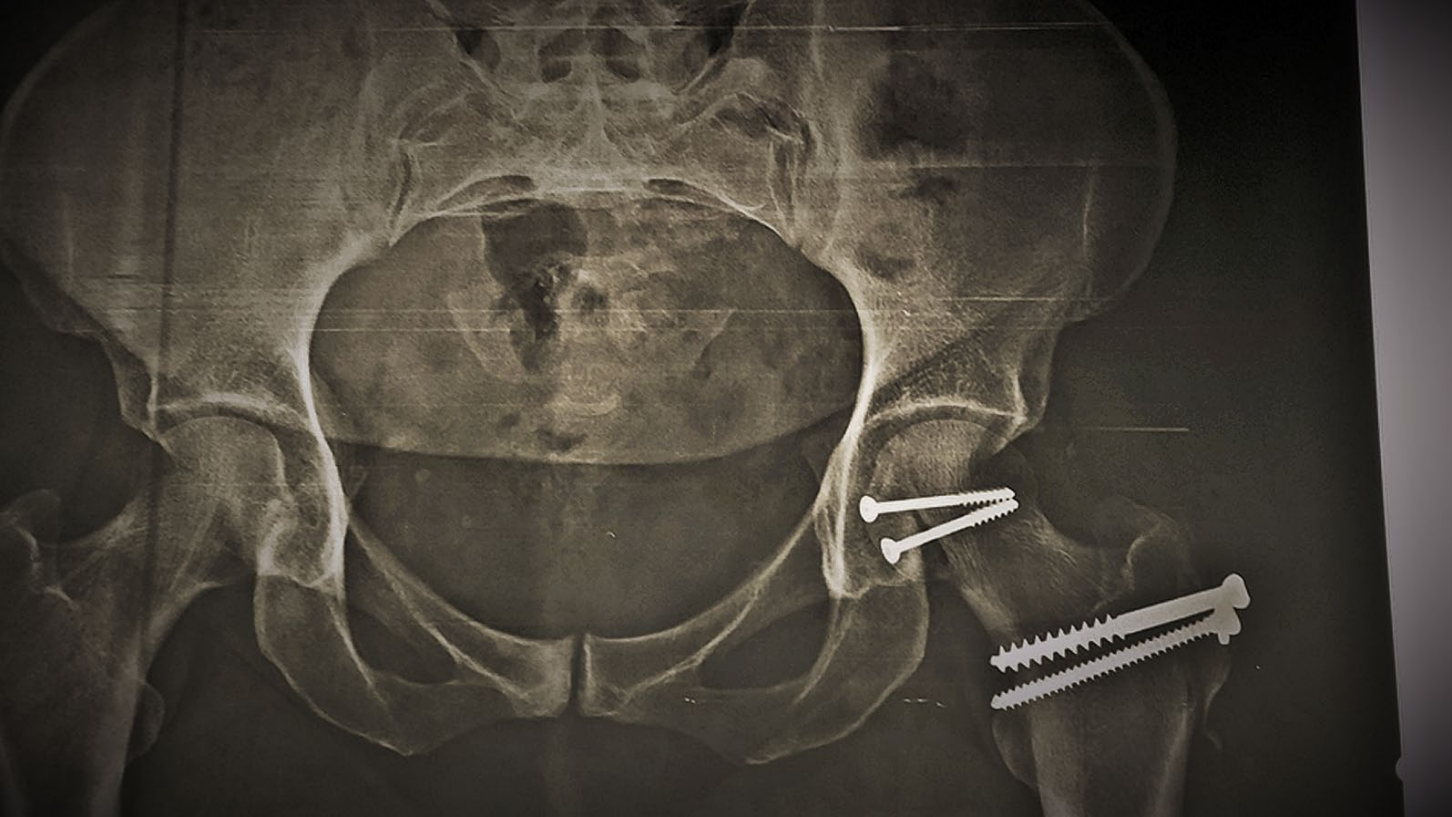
Follow-up radiograph at 1 month postoperatively
